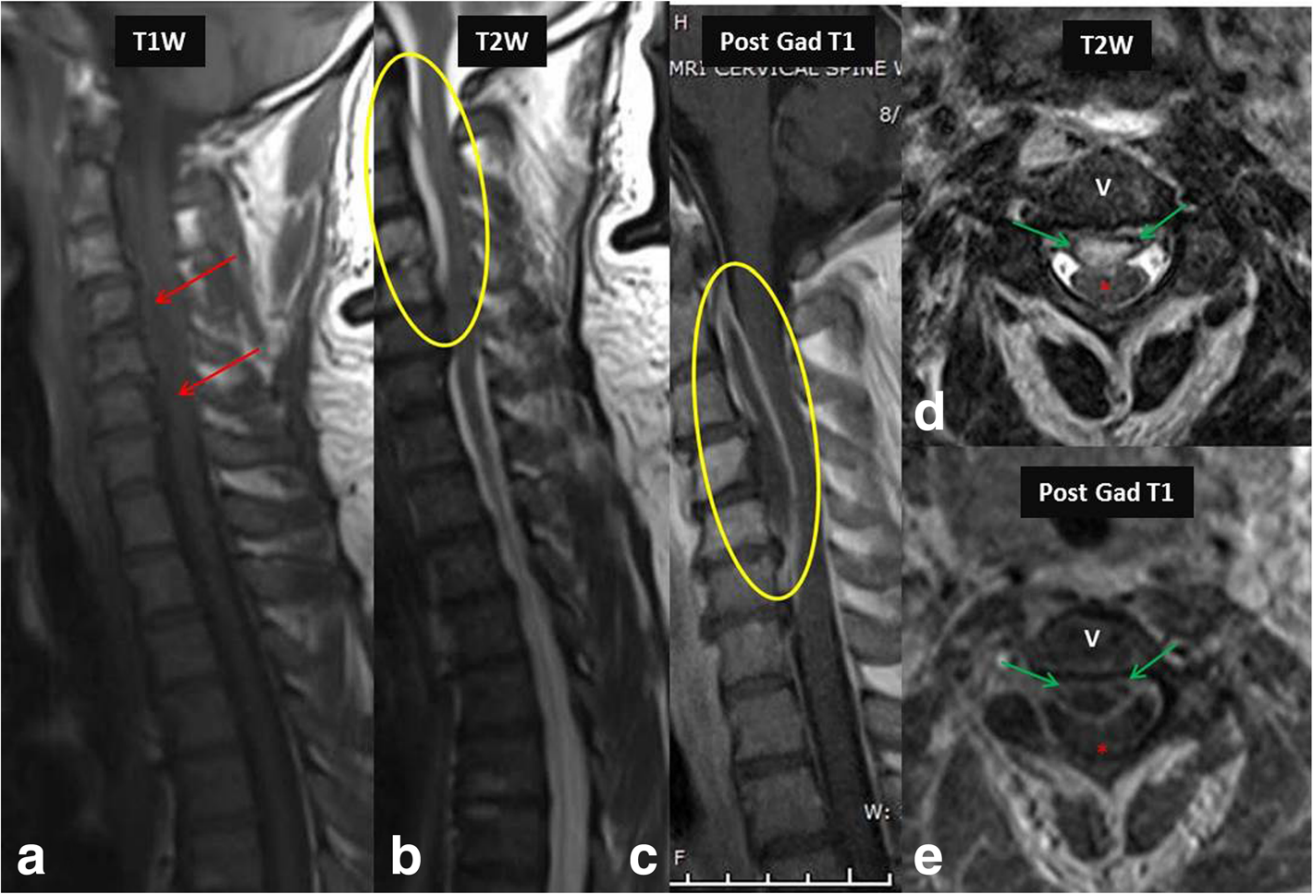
MR imaging in a 42-year-old female with paraparesis shows heterogeneous signal (*red arrows*) on T1 W image (a) and hyperintense epidural collection (*yellow oval*) on T2 W image (b) and show rim enhancement (*yellow oval*) on post contrast images (c), consistent with an epidural abscess. Axial T2 W (d) and post contrast images (e) distinctly show the epidural abscess (*green arrows*) causing indentation and posterior displacement of the thecal sac and cord (*red asterisk*). *Escherichia coli* was the causative organism. V- Vertebral body
